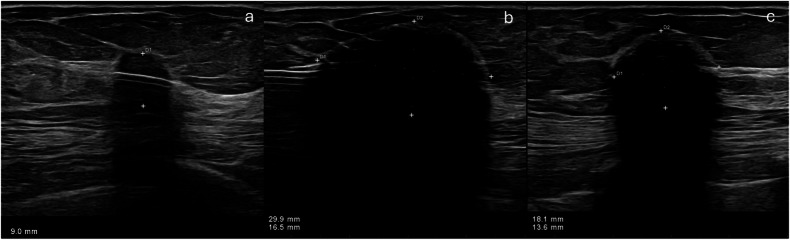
Ultrasound-guided cryoablation of 9-mm cancer in the outer upper quadrant of the left breast. a The transversal image represents the cryo-probe inside the core of the target lesion. b The transversal image demonstrates the “ice ball” formation, during the first cryoablation freeze cycle, which completely encompasses the tumor. c The image shows the reduction in size of the “ice ball” during the first thaw cycle
